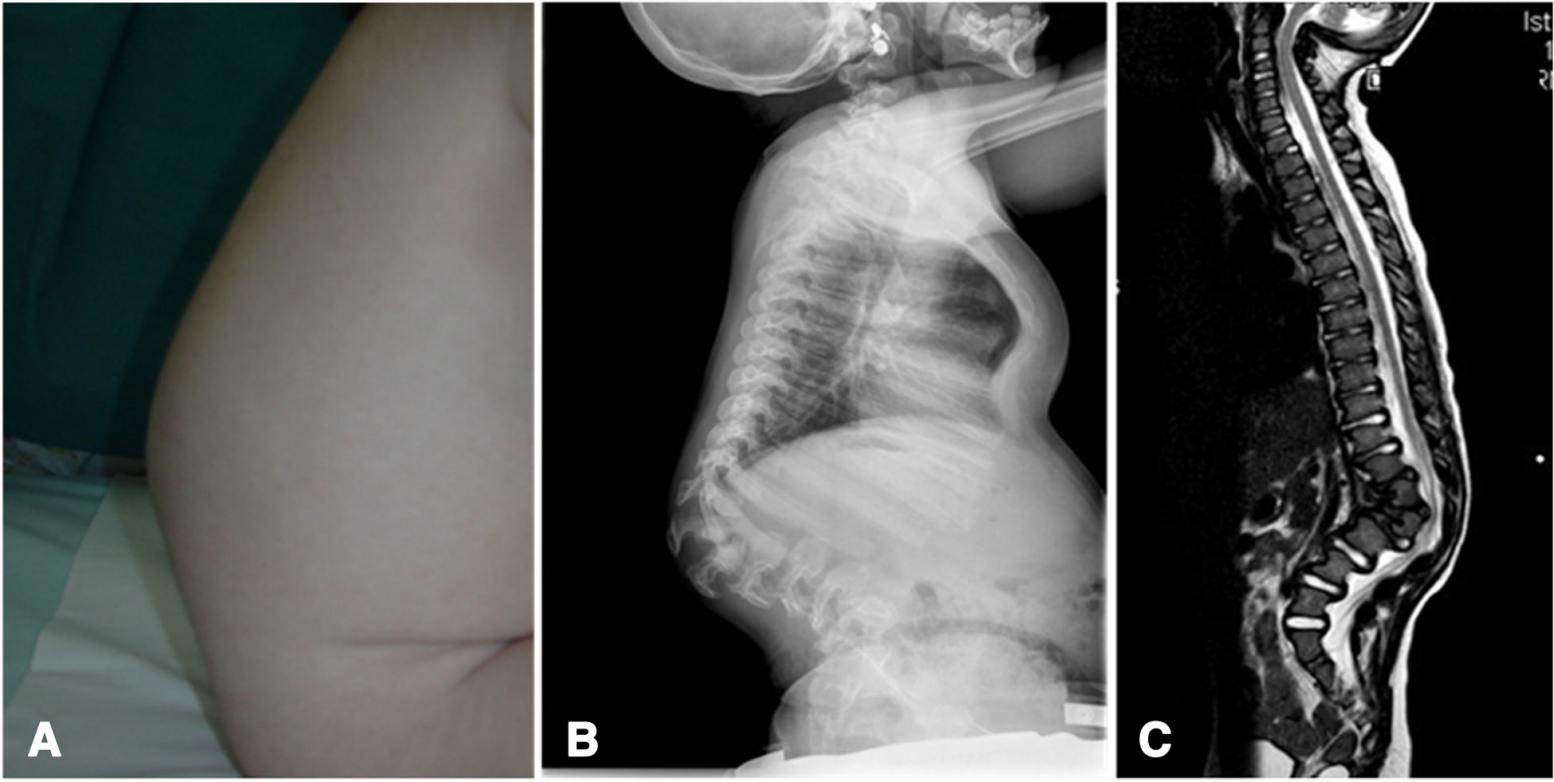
MPS IH. a Gibbus in a 9-month-old patient. b Spine x-ray of the spine in a 3-year-old patient. c T2-weighted magnetic resonance image of the spine showing significant kyphosis and vertebral canal stenosis
